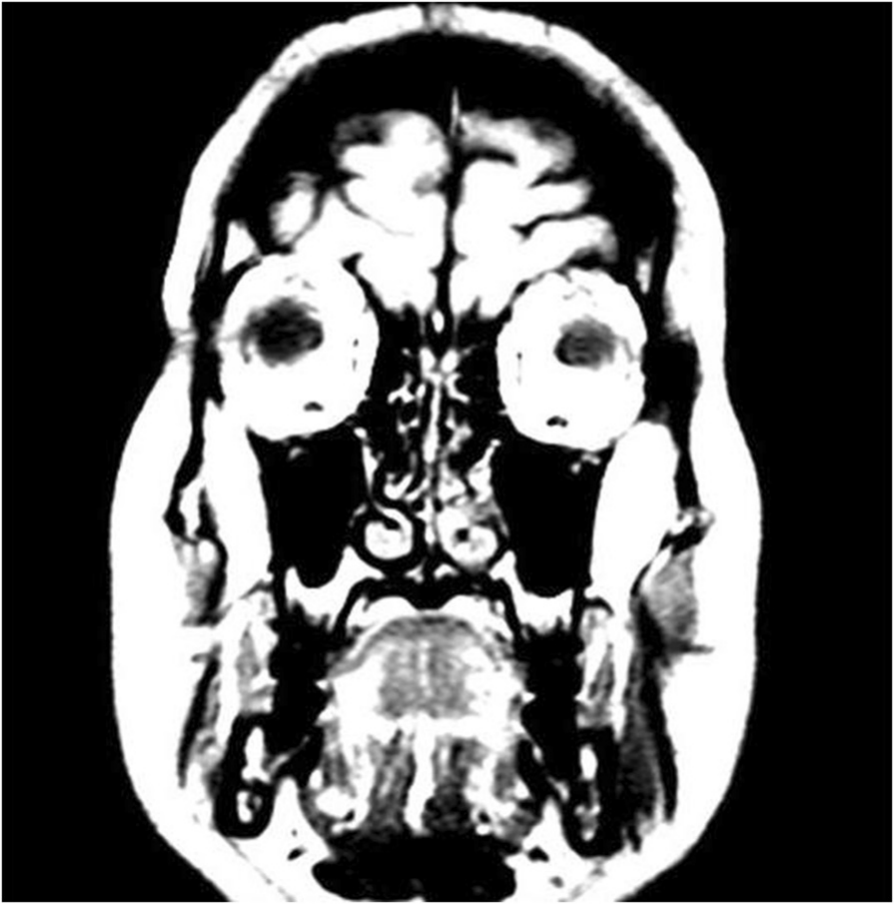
Magnetic resonance imaging displaying capacious cerebral spinal fluid spaces around the optic nerves.
